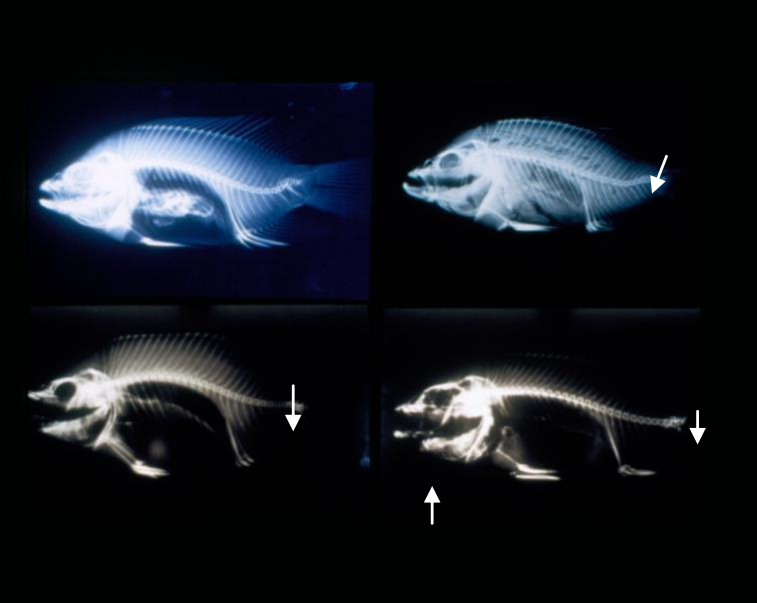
Different Types of Skeletal Deformities in Tilapia. Upper left: X-ray of tilapia collected from Lin-Bien River in October, 1994. Arrow shows vertebral segments close to tail are fused, calcified and deformed. Lower left: X-ray of tilapia collected from TKS station of Tongkong River in October, 1994. Note fusion of vertebrae near the tail (arrow). Upper right: X-ray of tilapia collected from the mouth of the Tongkong River in May, 1995. Note lordosis near the tail (arrow). There was also muscular atrophy in the tail area as well. Lower right: X-ray of tilapia collected from Kao-Ping River in October 1994. Arrow shows heavy calcification in the lower jaw, and fusion of vertebrae near the tail.
